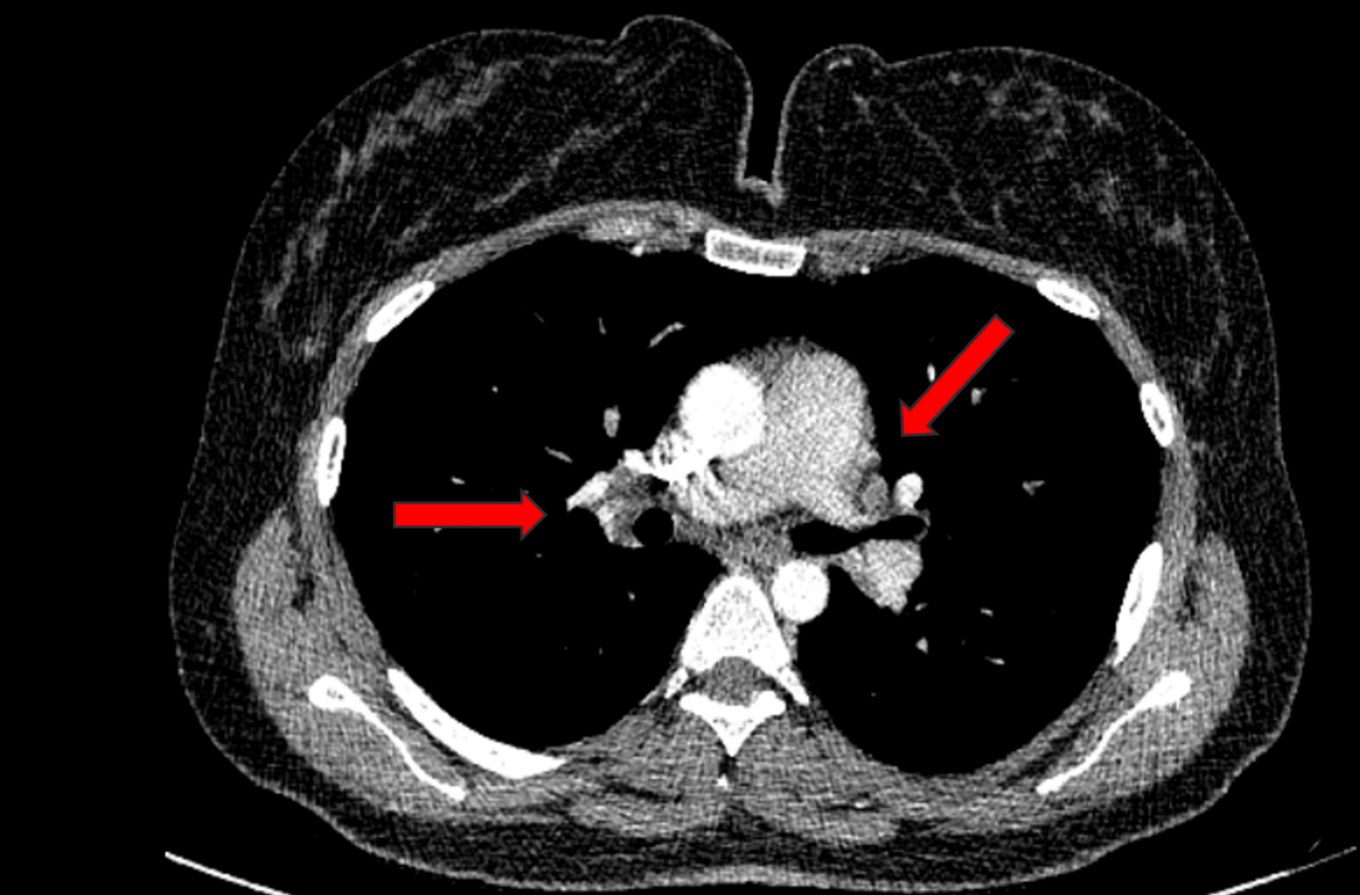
Repeat CT of the chest 11 weeks post-initiation of upadacitinib showing the near-resolution of hilar lymphadenopathy CT: computed tomography
